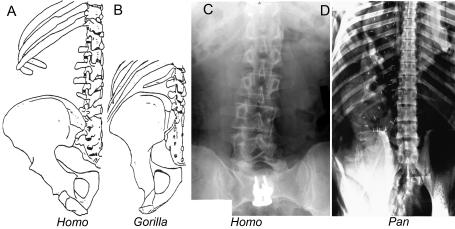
Secondary sacral boundary shifts within the hominiform clade. (A) Humans appear to retain the original hominiform longer flexible lumbar region. (B) Anatomical reconfiguration results in effective elimination of the lumbar region in *Gorilla*. (C) Despite a modal number of 5 lumbars, humans may have 4 lumbar but maintain a long flexible lumbar region. (D) Molecular phylogeny suggests that lumbar region shortening in *Pan* occurred independently and convergently (X-ray in D after Filler 1979 [92]).
